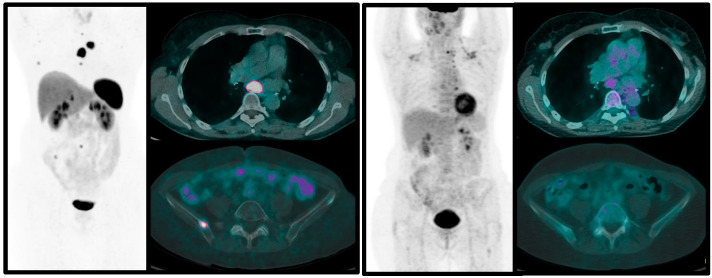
Sixty-three-year-old female patient with Atypical PC (Ki-67 20%). The combined imaging with [^18^F]FDG (left) and ^68^Ga-DOTA-NOC PET/CT (right) show high glucose metabolism of the lesions and faint somatostatin receptor expression, suggesting an aggressive histotype.
